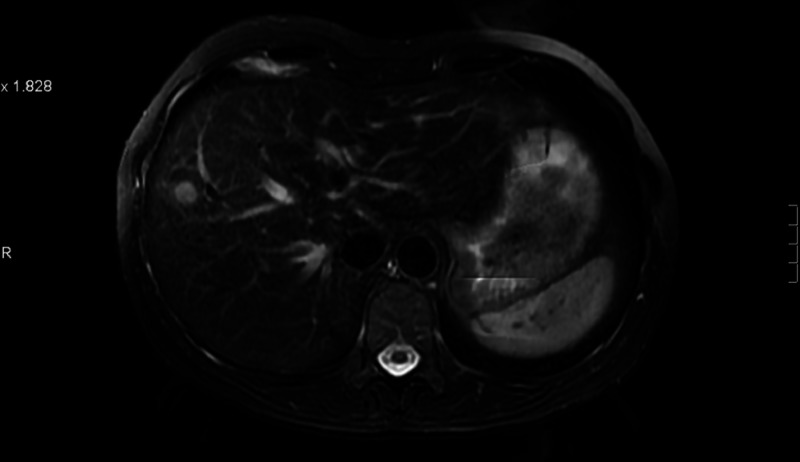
Patient 3: Magnetic resonance imaging (MRI) liver demonstrates single liver metastasis pre treatment
